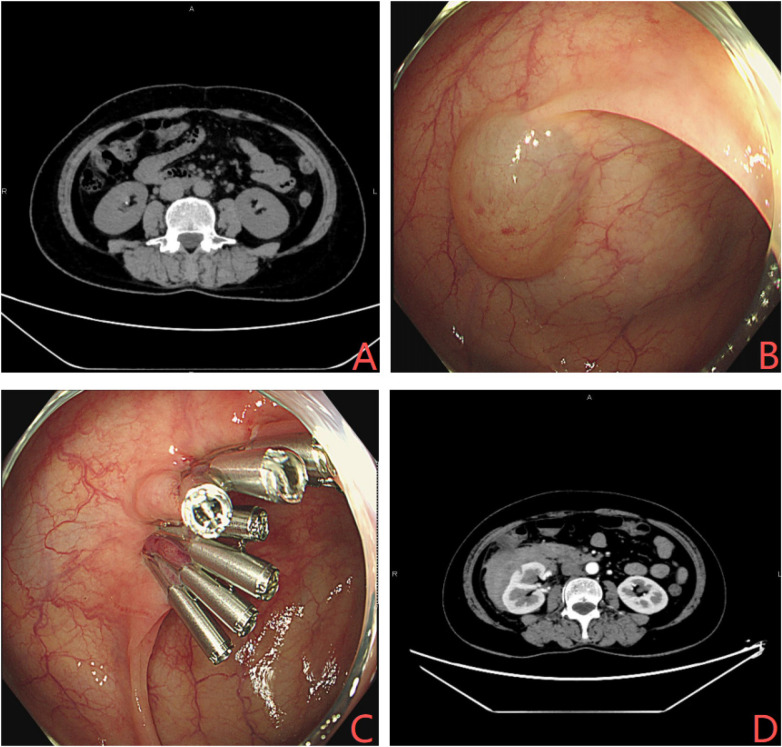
Imaging and procedural findings in a 50-year-old woman with right kidney contusion following EMR for hepatic flexure lymphangioma. (A) CT images of the chest and upper abdomen show liver cysts and small kidney stones in the right and left kidney. (B) Colonoscopy image shows 3 cm cystic mass within the hepatic flexure suggestive of lymphangioma. (C) Intraoperative image showing repeated electrocoagulation and titanium clamp used for resection of lesion. (D) Postoperative contrast-enhanced CT image shows ascending colon wall edema, pericolic free gas, perirenal hematoma, and hemorrhagic focus in the right kidney cortex suggestive of right kidney contusion.
